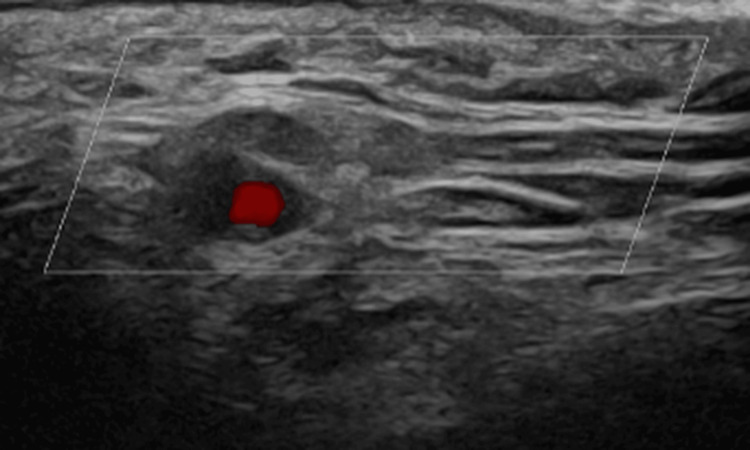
Ultrasound temporal artery scan. Halo sign in left common temporal artery, in keeping with giant cell arteritis (GCA)
